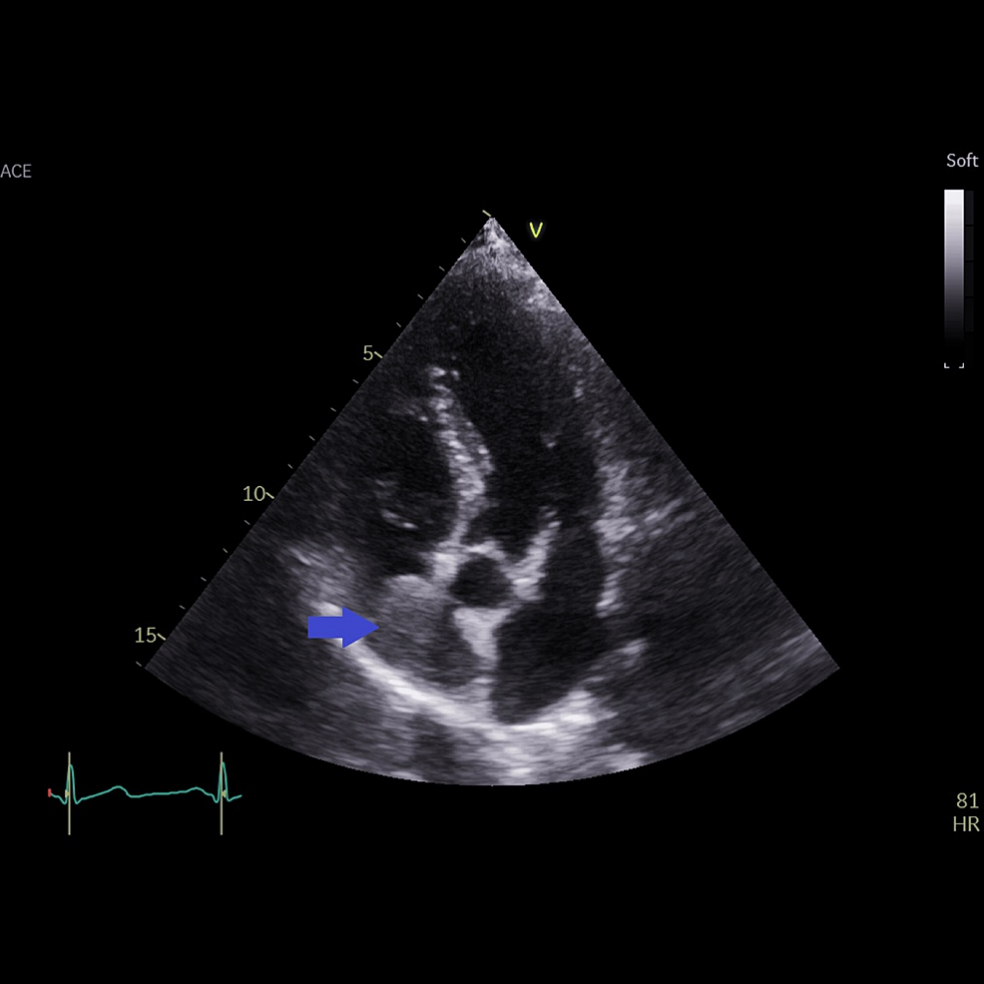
Apical five-chamber view showing the right atrial mass (blue arrow); the origin or the attachment of the mass could not be visualized from this view. The lower (juxta-atrial) superior vena cava could not be visualized from this view.
